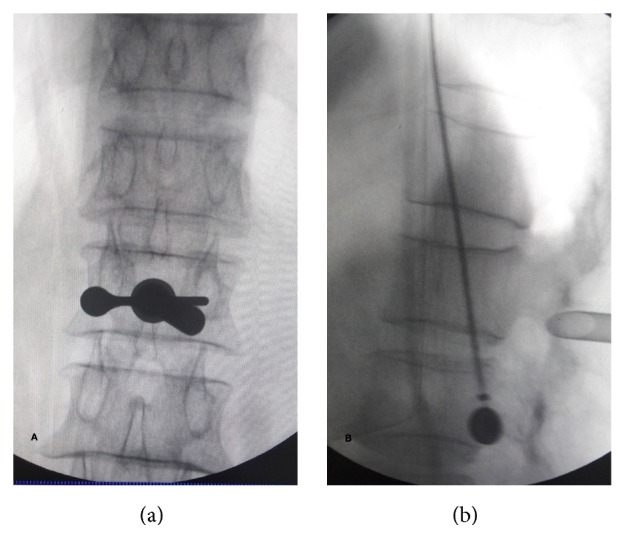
Intraoperative C-arm images of the procedure. Insertion of the working sheath toward the right lower laminar of the lumbar 1 vertebra. Anteroposterior radiograph showing the downward inclination of the working sheath lying at the medial pedicular line (a). Lateral radiograph showing the working sheath piercing the lamina (b).
